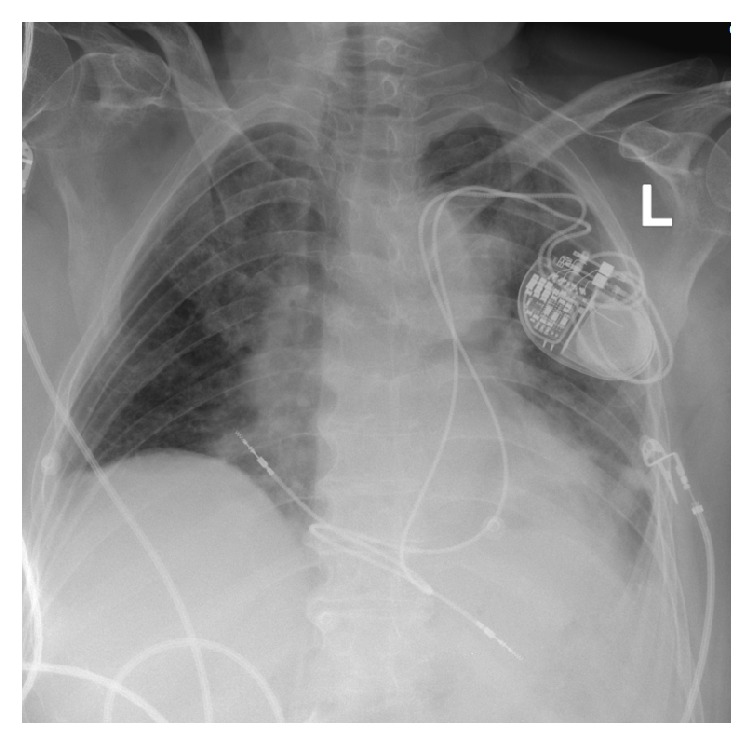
Chest X-ray showing final position of pacemaker and leads.
